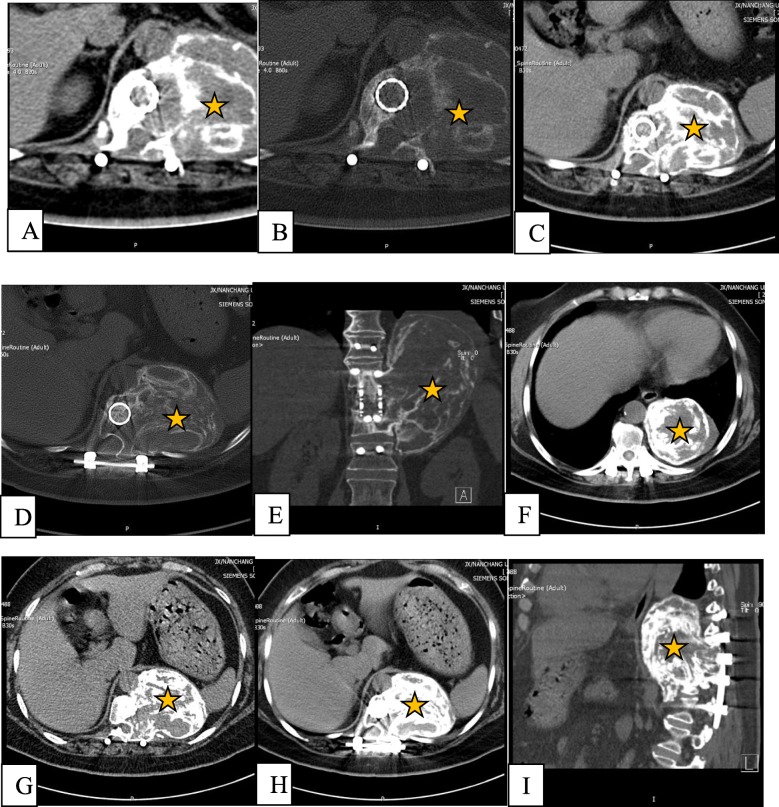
CT scan of the thoracolumbar spine after denosumab therapy. Images a and b were obtained in July 2015; images c, d, and e in November 2015; and images f, g, h, and i in June 2016. These images show the calcification of the tumour and shrinking after denosumab therapy
